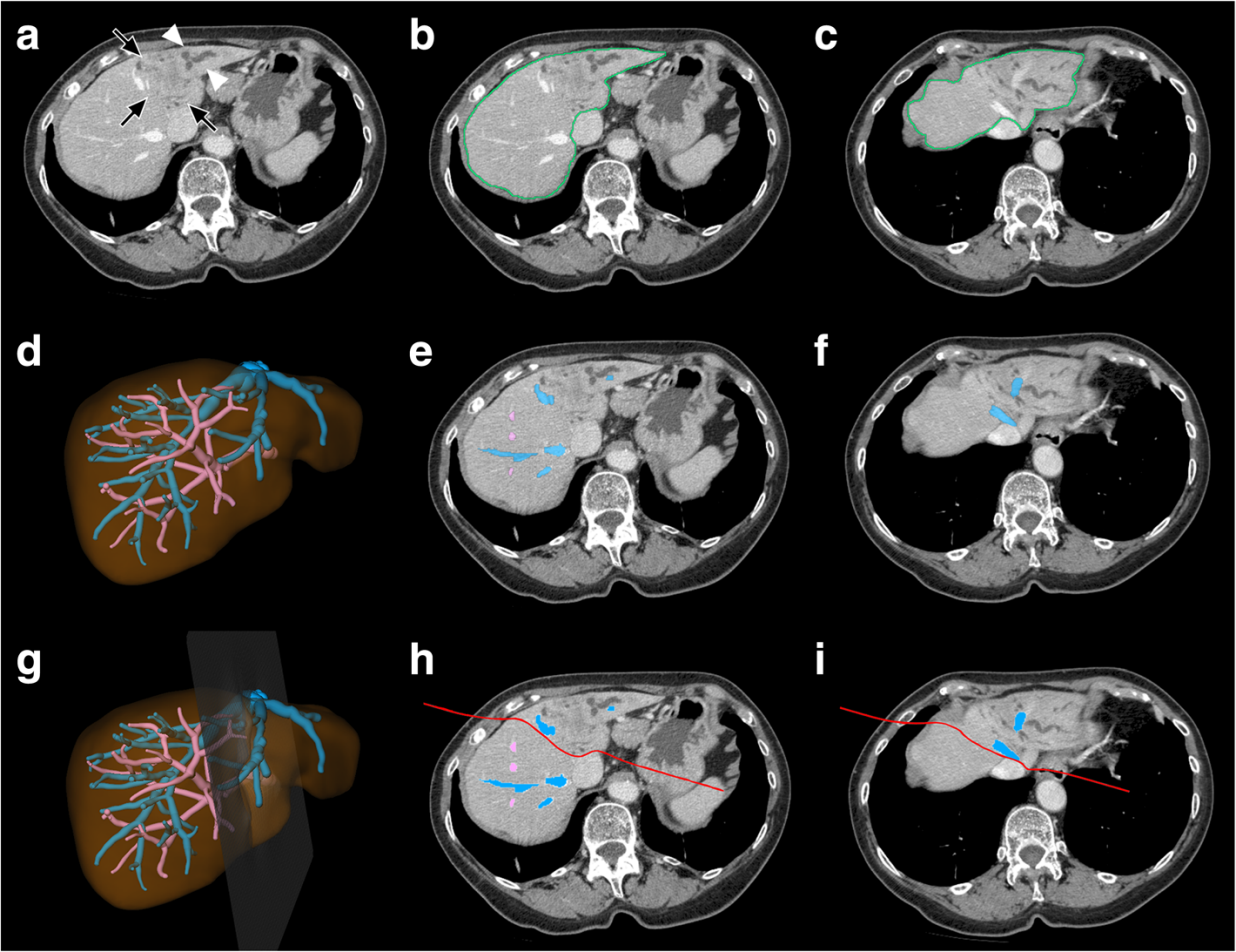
Segmentation process in a patient planned for left hemihepatectomy with resection of the MHV due to intrahepatic CC a) Plain axial CT image at the level of the maximum tumor extension shows the hypoattenuated tumor in the atrophic left liver lobe (*black arrows*) with upstream cholestasis (*white arrowheads*). b) and c) Detection of the liver outline. d) – f) Detection of the intrahepatic PV (*in pink*) and HV (*in blue*). Note occlusion of the left PV and of branches of the MHV and left HV due to tumor invasion. g) – h) Definition of the transection plane (*in red*)
